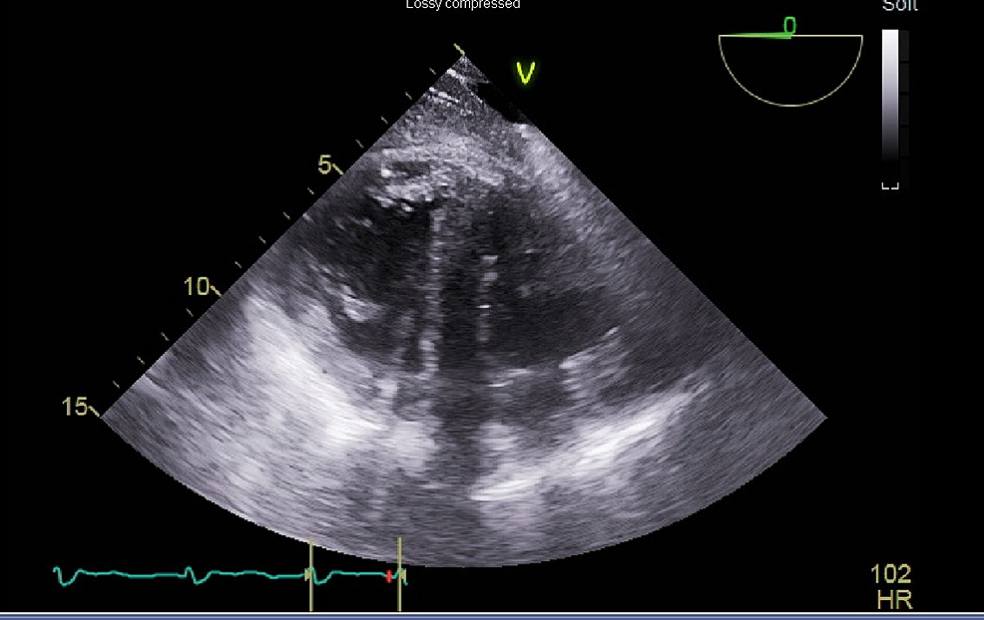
Transesophageal echocardiogram transgastric short-axis view shows improved right ventricular size after separation from the cardiopulmonary bypass machine.
